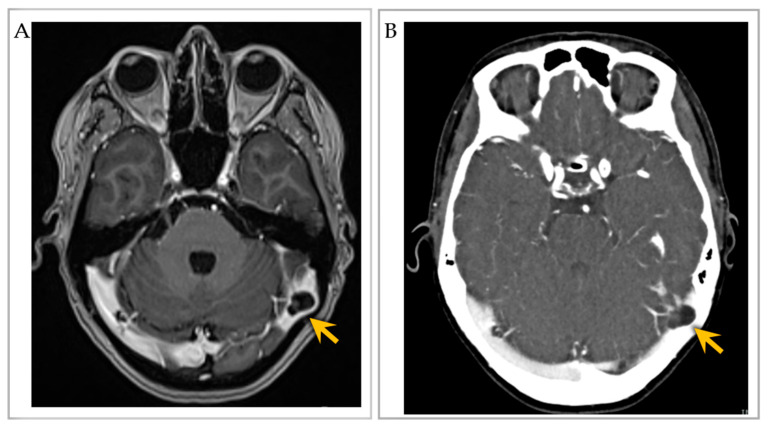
Imaging of an individual with multiple intrasinus-type GAGs. (A) Post-contrast T1-weighted brain MRI axial image shows two GAGs along the right and left lateral transverse sinuses, with severe sinus narrowing on the left (arrow). (B) CT venogram axial image further depicts the two GAGs with severe left lateral transverse sinus luminal narrowing (arrow). Images reproduced from *Int. J. Mol. Sci.* 2023, *24*, 11410 [45].
